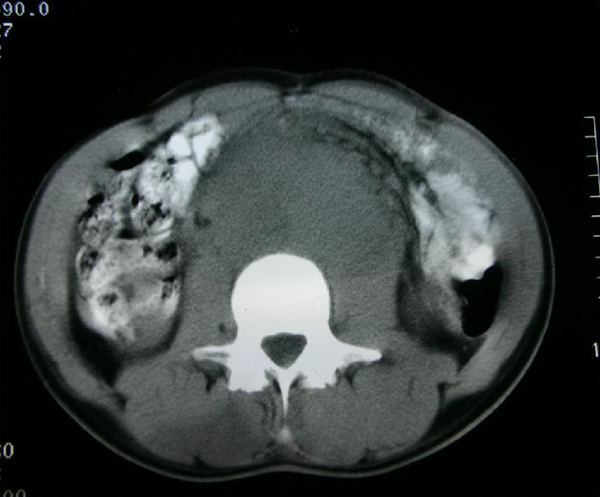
Abdominal computed tomography images revealing a large retroperitoneal mass, extending across the midline.
